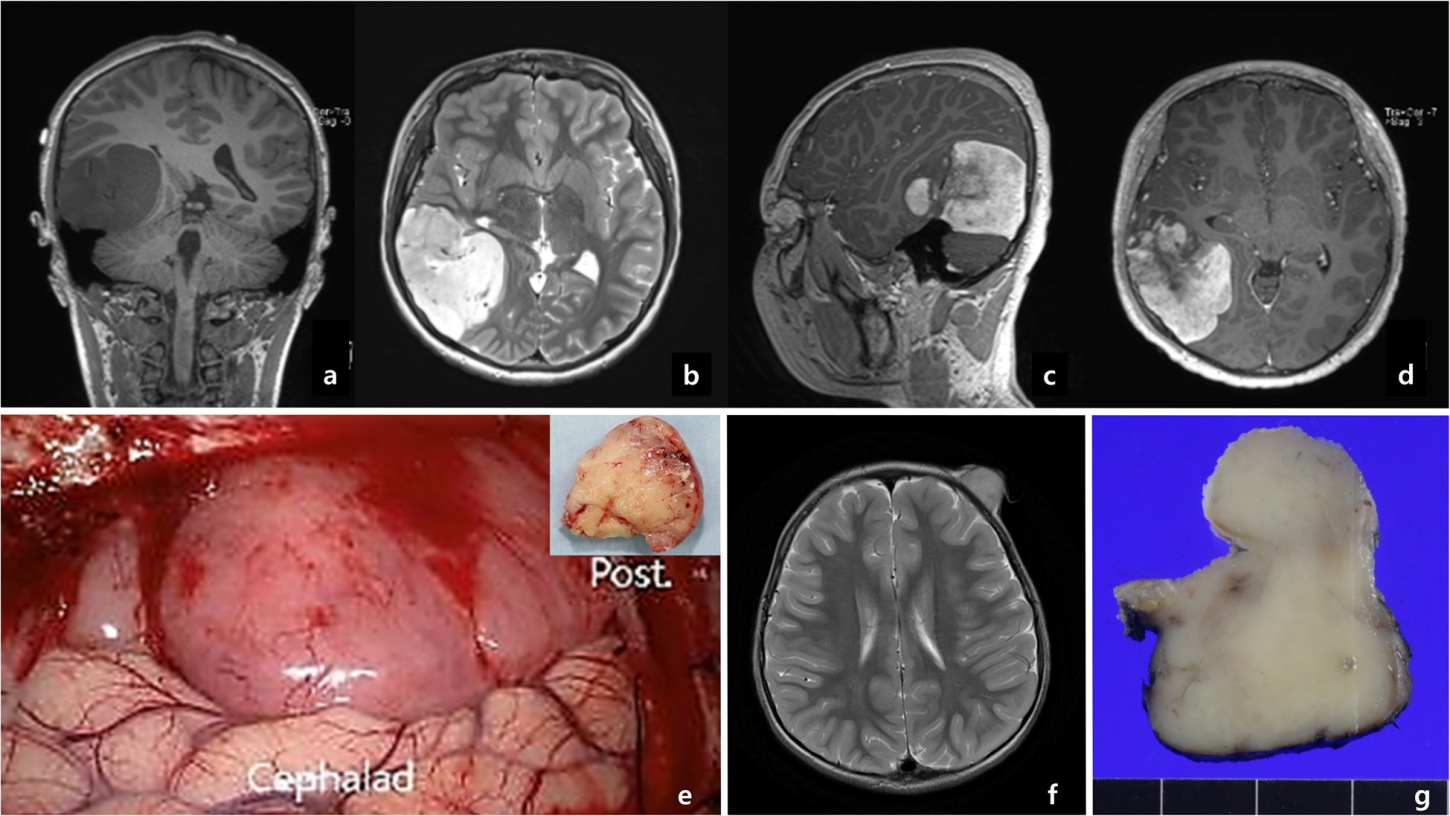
a-e Case 1 with *TPR*-*NTRK1* fusion.: MRI reveals a) T1-low, b-d) T2-high dura-based mass with enhancement. e The tumor was located in the right temporal convexity and right cerebellar tent (The direction of the brain mentioned as cephalhead and posterior). The inlet is the cut surface of the tumor showing yellowish, and solid without hemorrhage or necrosis. f, g Case 2 with *LMNA-NTRK1* fusion tumor: T2 weighted MRI revealed low-density mass on the left forehead. The cut surface of the tumor shows a gray-white solid appearance without hemorrhage or necrosis
